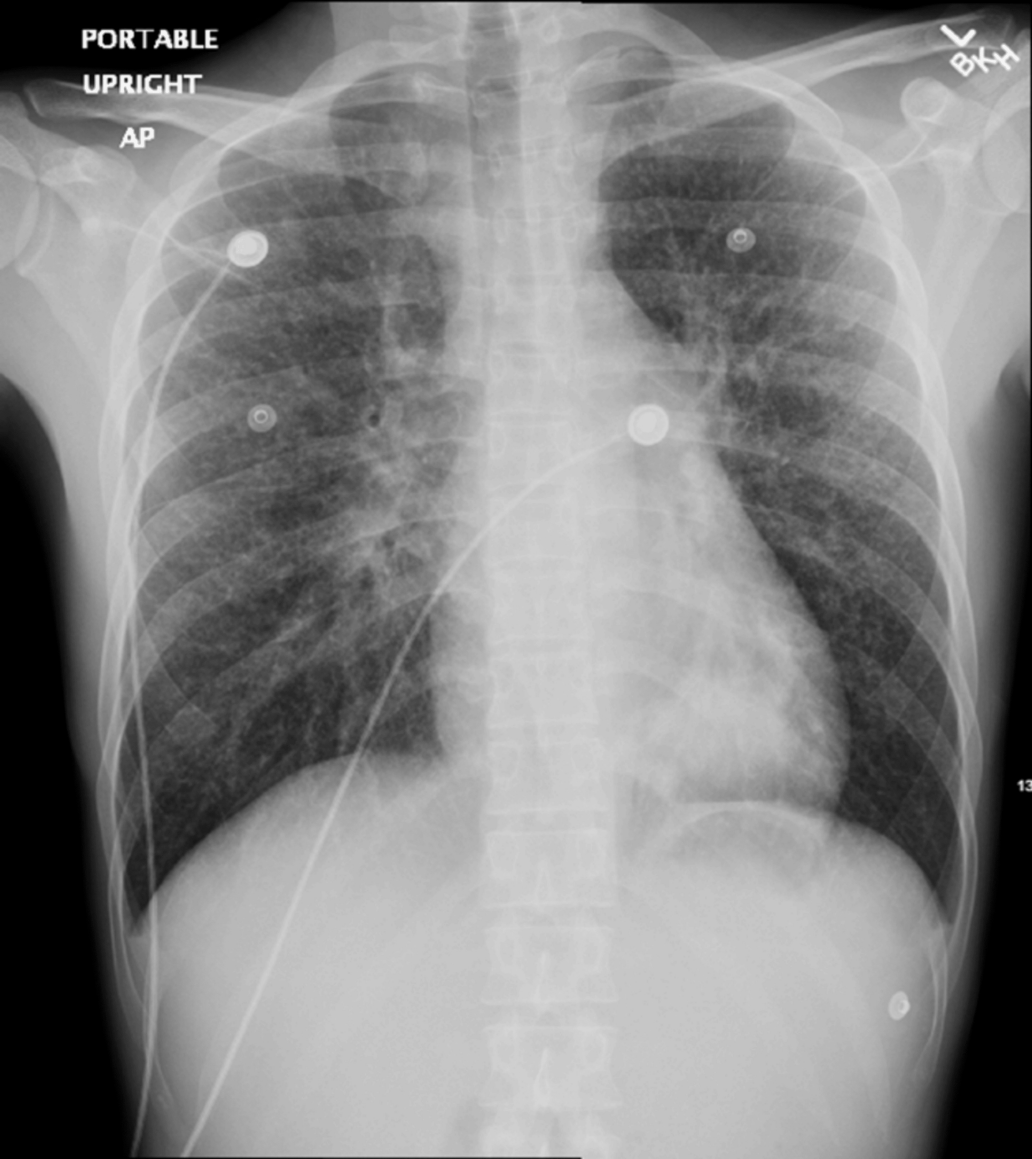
Initial chest X-ray showing perihilar suprahilar miliary nodules, extensive bilateral tiny nodular infiltrates, left lower lobar atelectasis consolidation, and hilar prominence
